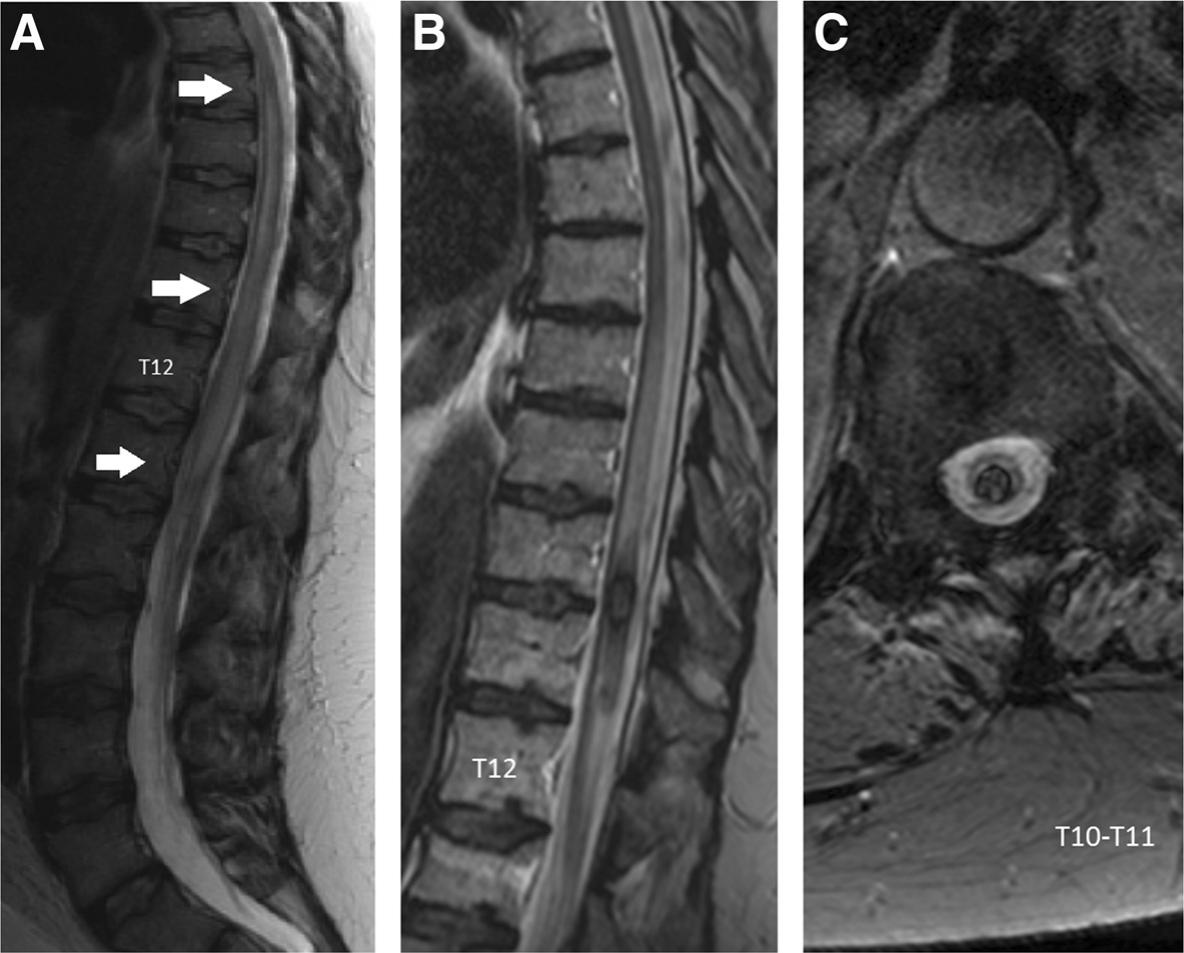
Spinal MRI images of the first inflammatory event (a) and the hematomyelia (b and c). a. Sagittal T2-weighted MRI showing a high-intensity signal and enlargement of the spinal cord extending from T8 to the L1-L2 level. b. Sagittal T2-weighted turbo spin-echo MRI showing a heterogeneous low-intensity signal at the T10-T11 level. c. Transversal T2*-weighted gradient-echo MRI at the T10–T11 level confirming the intramedullary low-intensity signal: it is an intramedullary hemorrhage (hematomyelia)
